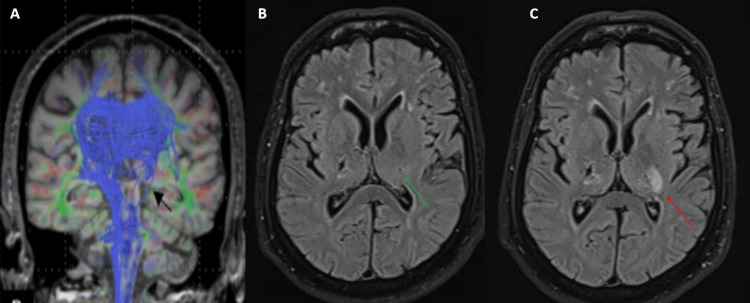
(A) Diffusion tensor imaging tractography showing disruption of left-sided corticospinal fibers (black arrow); (B) Brain MRI scan FLAIR sequence on first day of presentation showing hyperintensity in left capsule-ganglionic region (green arrow); (C) repeat imaging of the same region after four days showing lesion expansion (red arrow). MRI: magnetic resonance imaging; FLAIR: fluid-attenuated inversion recovery
